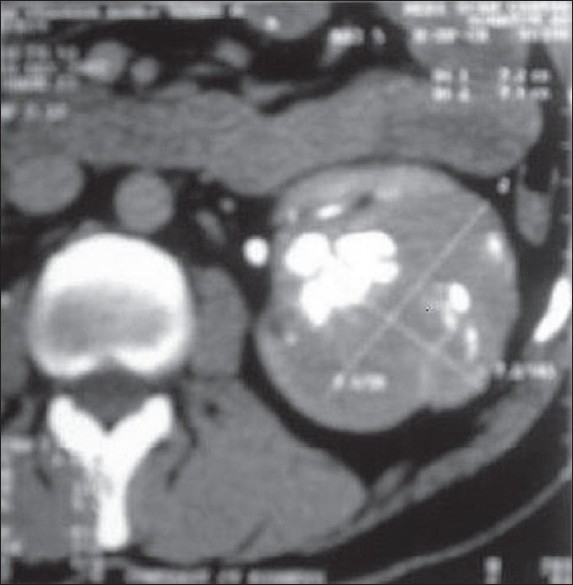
Computed tomographic scan of abdomen demonstrating left lower pole renal mass with areas of calcification
